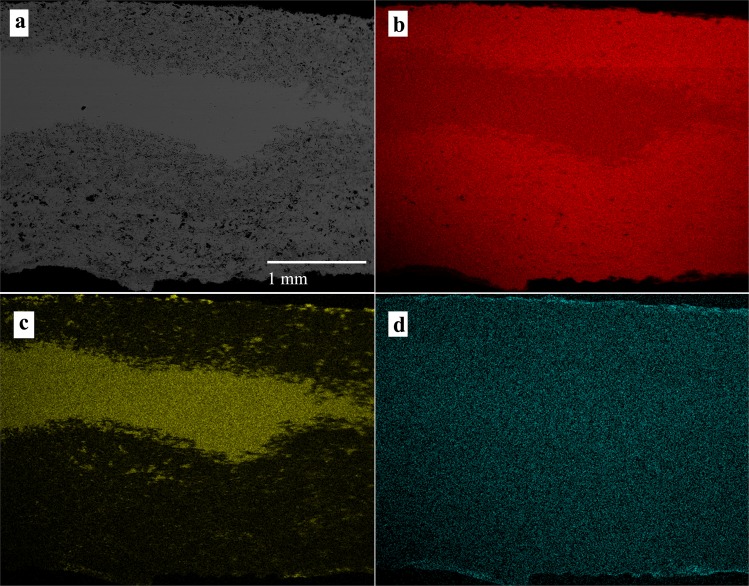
BSE image of sample 5# (a) and X-ray maps of Cu (b), Zn (c) and Fe (d).
